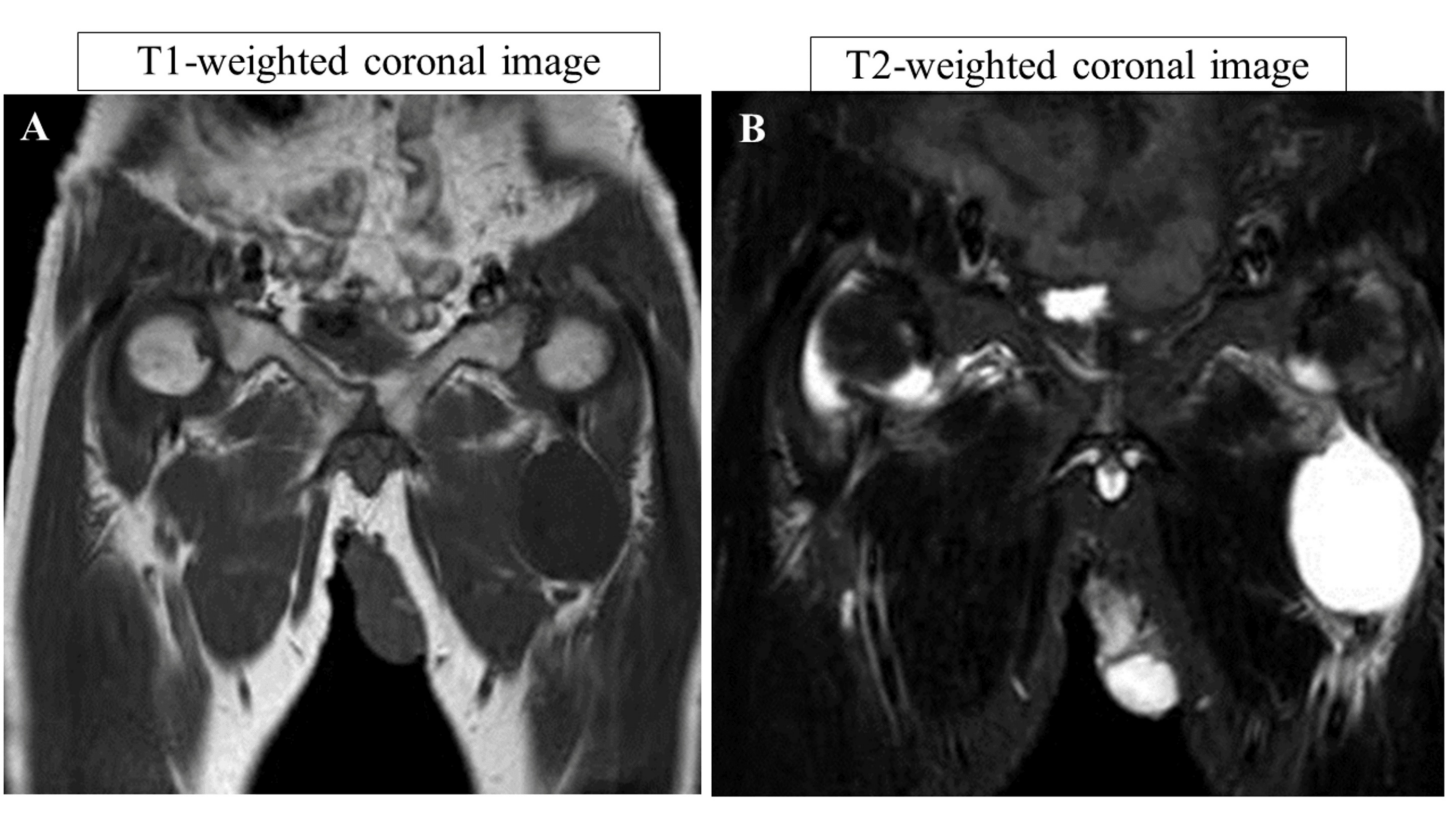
Preoperative imaging of the hip (MRI) Hip MRI scan of the hip synovial bursitis, primarily on the left side. (A) Low-intensity signal on the T1-weighted coronal image. (B) High-intensity signal on the T2-weighted coronal image. MRI: magnetic resonance imaging
